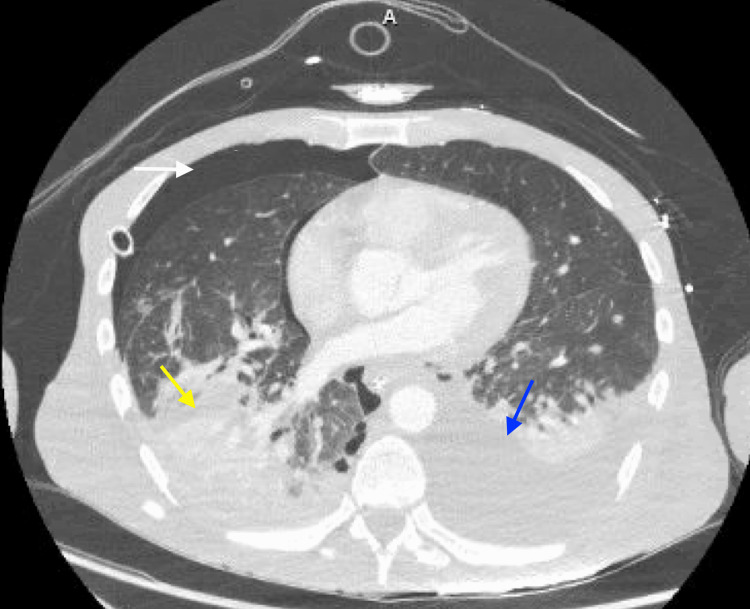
CT of the chest with IV contrast showing pneumothorax (white arrow), right posterior pneumonia (yellow arrow), and left pleural effusion (blue arrow).
